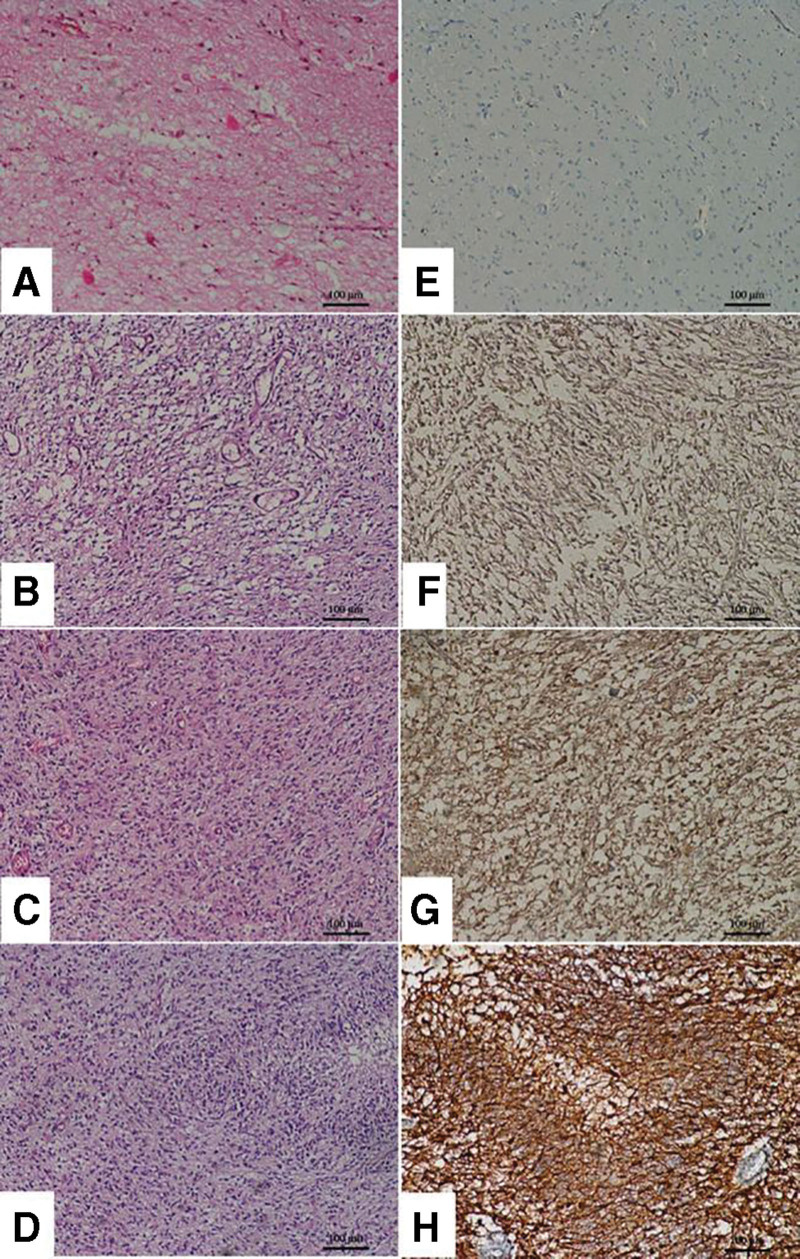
qRT-PCR results of UCH-L1 expression of astrocytoma tissues. A. 18S CT values, B. UCH-L1 CT values, C. Normalized UCH-L1 mRNA expression relative to control values. .
